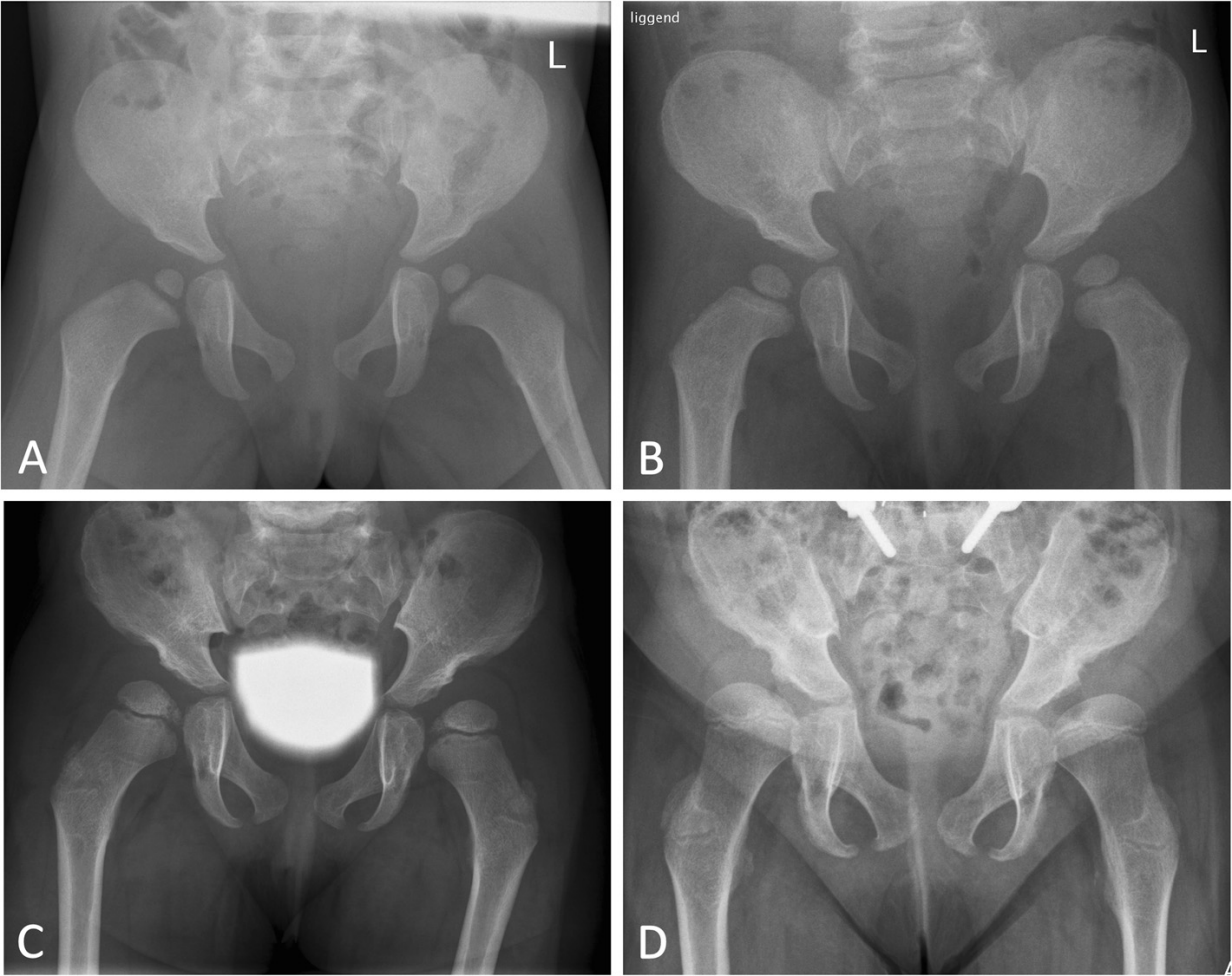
Sequential X-ray studies of a MPS I-H patient who underwent successful HSCT at the age of 2 yrs and 6 m as well as spinal fusion at the age of 7. Characteristic signs included acetabular dysplasia with a steep acetabular angle, interruption of Shenton’s line, characteristic medial thinning of the femoral head and coxa valga. A: at diagnosis, age 1 yrs 3 m B: at 2 yrs 4 m C: at 4 yrs 8 m D: at 8 yrs 5 m.
